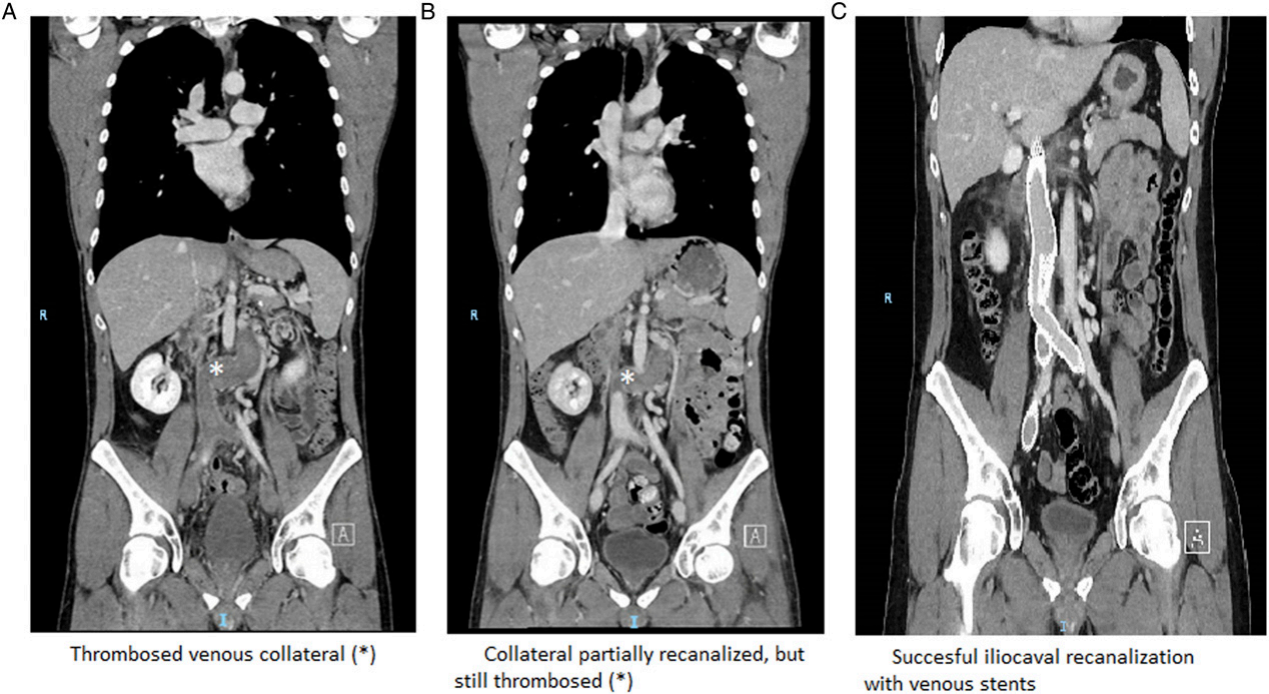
Computed tomography venography before thrombolysis (A); after thrombolysis (B); after venous stenting (C).
